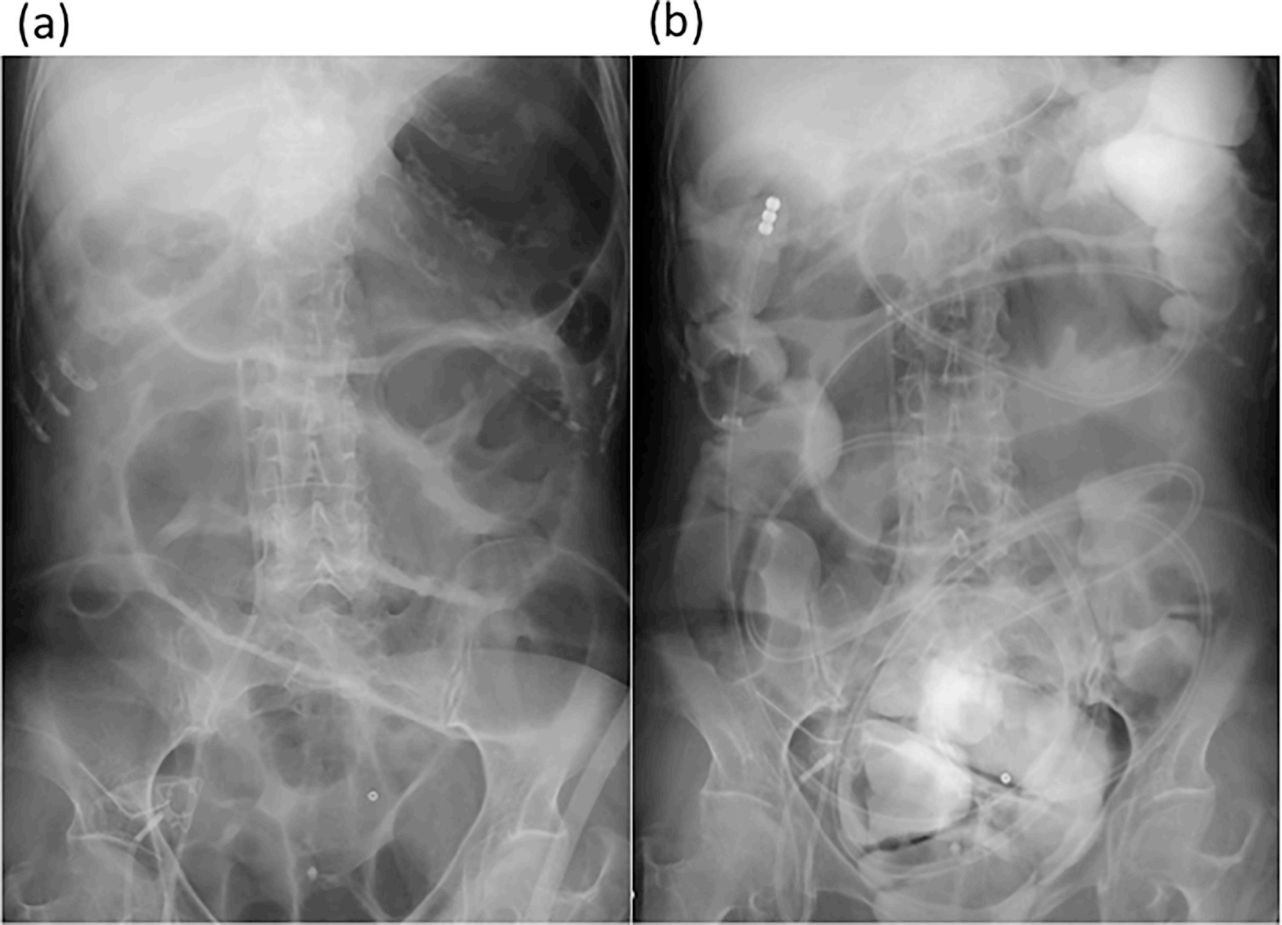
Radiological improvement after insertion of ileus tube. Abdominal radiograph on admission in a patient with adhesive small bowel obstruction (a) compared with the radiograph obtained 1 day after ileus tube decompression (b). The previous gas-filled or fluid-filled small bowel loops showed no evidence of distension, the air–fluid levels disappeared, and the tip of the ileus tube reached the colon.
